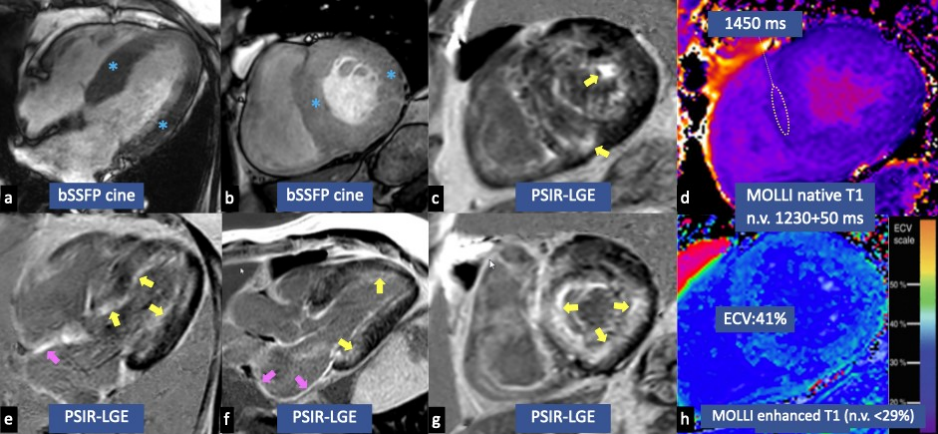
Cardiac magnetic resonance imaging (CMRI) findings of cardiac
